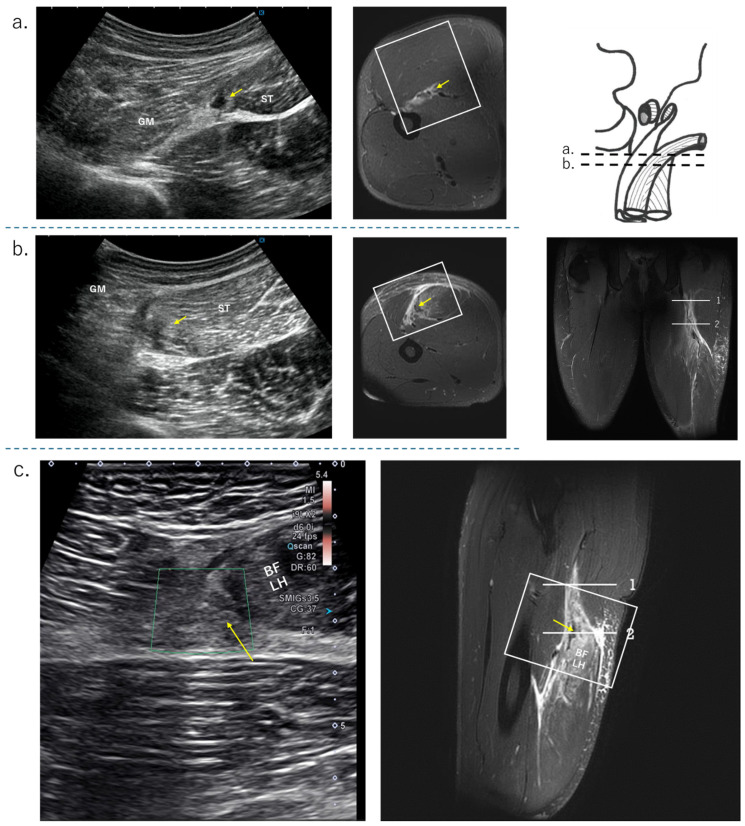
The US findings of the CT tear, MRI condition: T2-weighted image with fat suppression. (a) short-axis view at level 1, CT rapture and hematoma; (b) short-axis view at level 2, tortuosity CT (yellow arrow), and ST side hematomas, BFLH side hematomas; (c) long-axis view, CT dropped to the periphery (yellow arrow) due to CT rupture. Refer to the sagittal MRI image.
